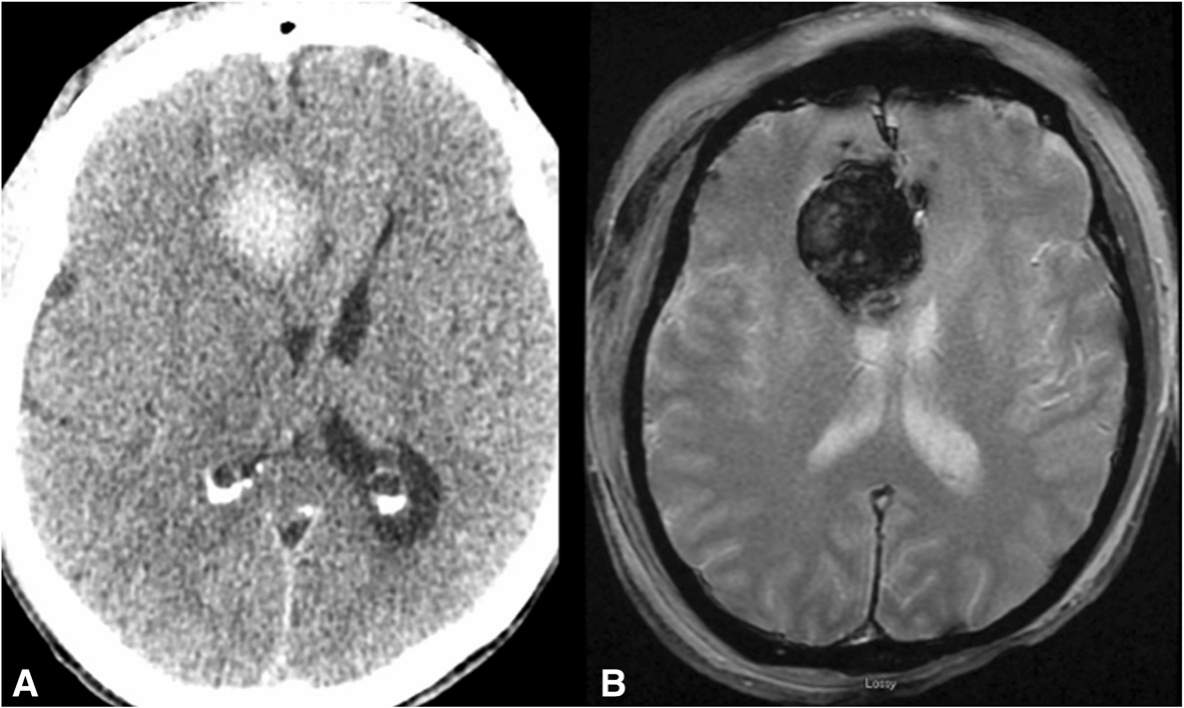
Postoperative imaging including computed tomography scan (A) and T2 axial (B) magnetic resonance imaging scan showing expansile postoperative hemorrhage.
